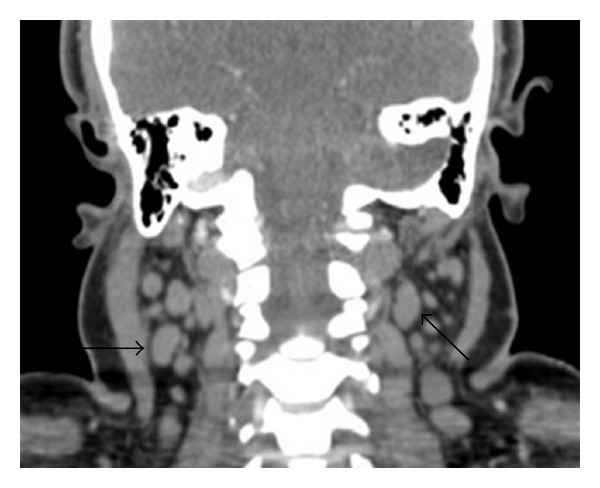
CT neck with contrast revealed diffuse cervical adenopathy (arrows demonstrate lymphadenopathy).
